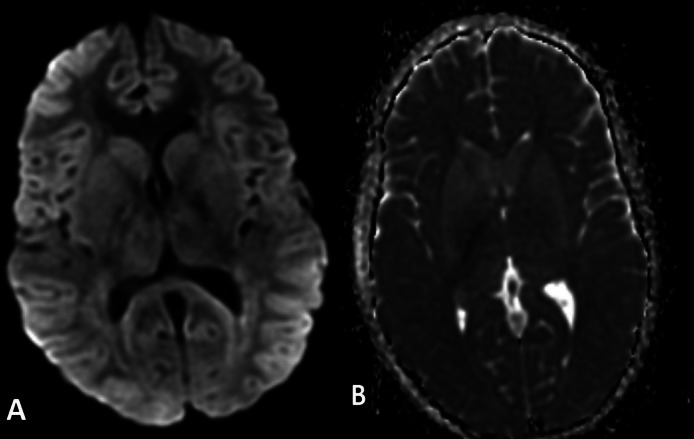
DWI sequence (A) with ADC (B) showing no restricted diffusion.
